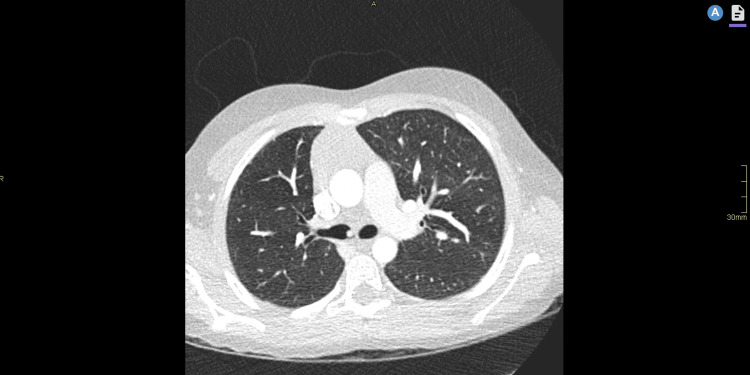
CT post debulking with a tiny enhancing stalk of residual tumour in the proximal right main bronchus
